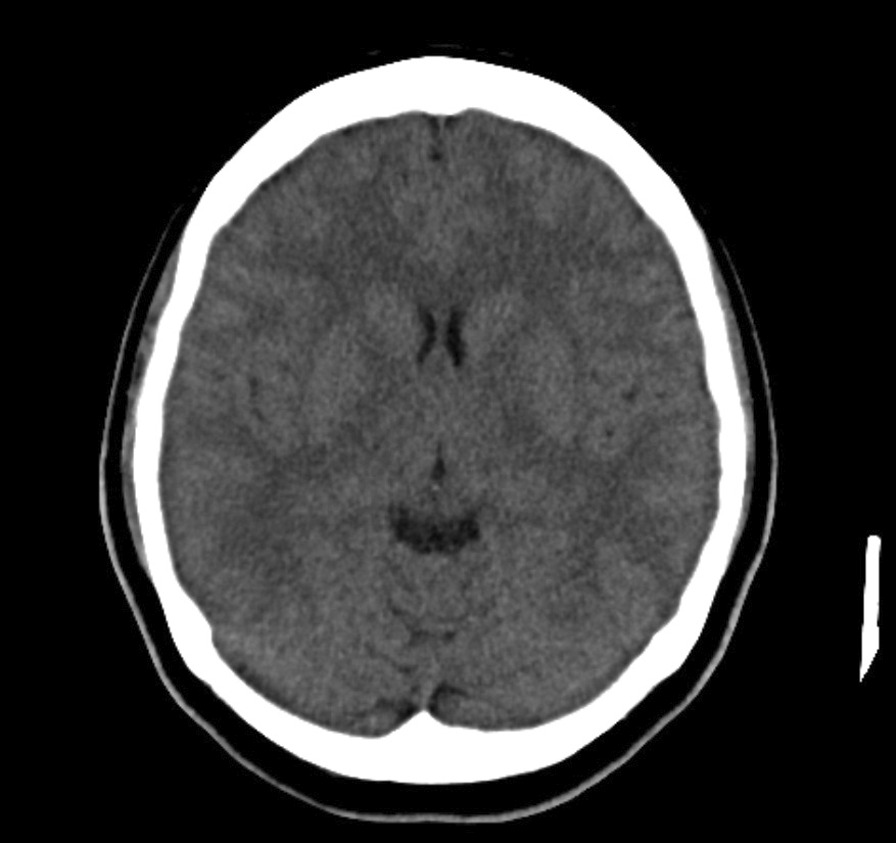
Computed tomographic imaging of brain on admission, showing no intracranial bleeding or space-occupying lesion
